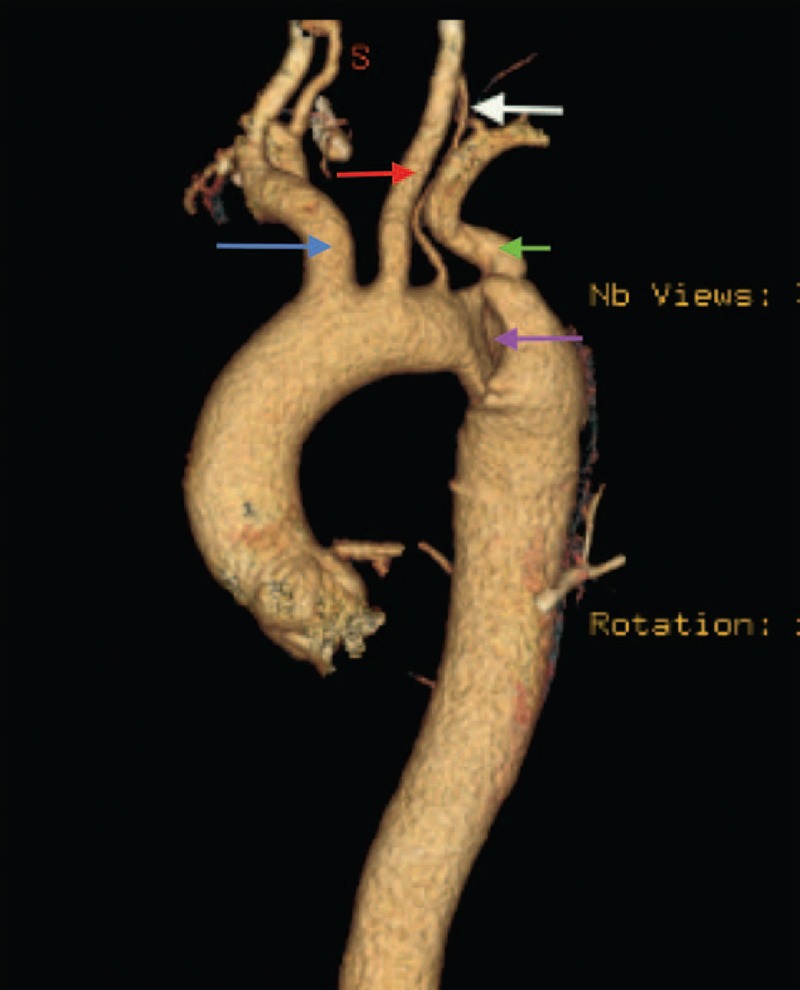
CT scan image (volume rendered). Aortic arch with vertebral pattern and Stanford B aortic dissection. (Blue arrow, brachiocephalic trunk; red arrow, left common carotid artery; white arrow, vertebral artery; green arrow, left subclavian artery; purple arrow, aortic dissection.) CT = computed tomography.
